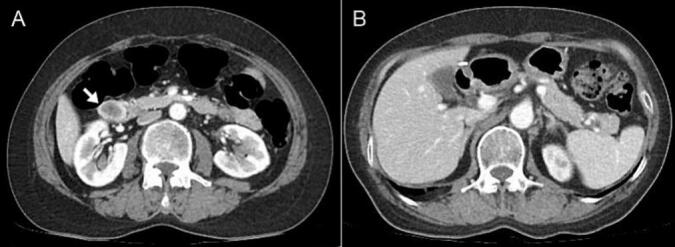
Abdominal computed tomography revealing a 2.7 cm sized inhomogeneous enhancing mass with central necrosis in duodenal 2nd portion without enlargement of the surrounding lymph nodes (A). Six months later, there were no abnormalities or recurrences in the postoperative follow-up computed tomography (B).
